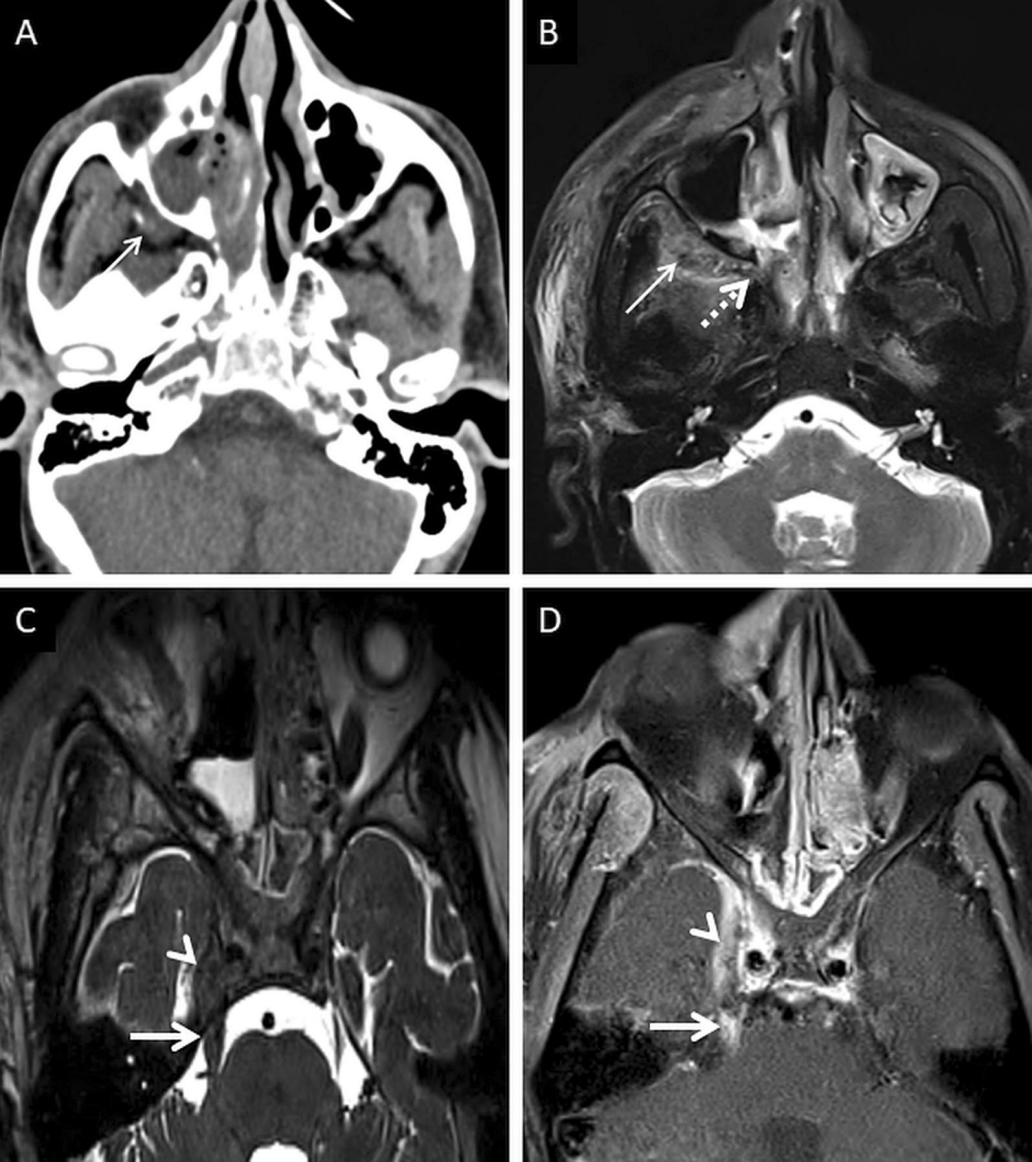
Post-operative follow-up demonstrating intracranial involvement in a case of ROCM. Axial CT image (a) of the neck reveals mild soft tissue in the right retro-antral (thin arrow). Axial T2-FS image (b) confirms the soft tissue occupying the right retro-antral fat (thin arrow) and PPF (dotted arrow). Axial constructive interference in steady state (CISS) sequence (c) obtained for the evaluation of the cranial nerves demonstrates thickening of the right trigeminal nerve (thick arrow). Note the lateral convexity of the right cavernous sinus (arrow head). CE FS T1W image (d) reveals dural thickening and non-enhancement of the right cavernous sinus in keeping with thrombosis (arrow head). Enhancement of the cisternal portion of the right trigeminal nerve (thick arrow in d) is also seen, suggestive of intracranial perineural spread
